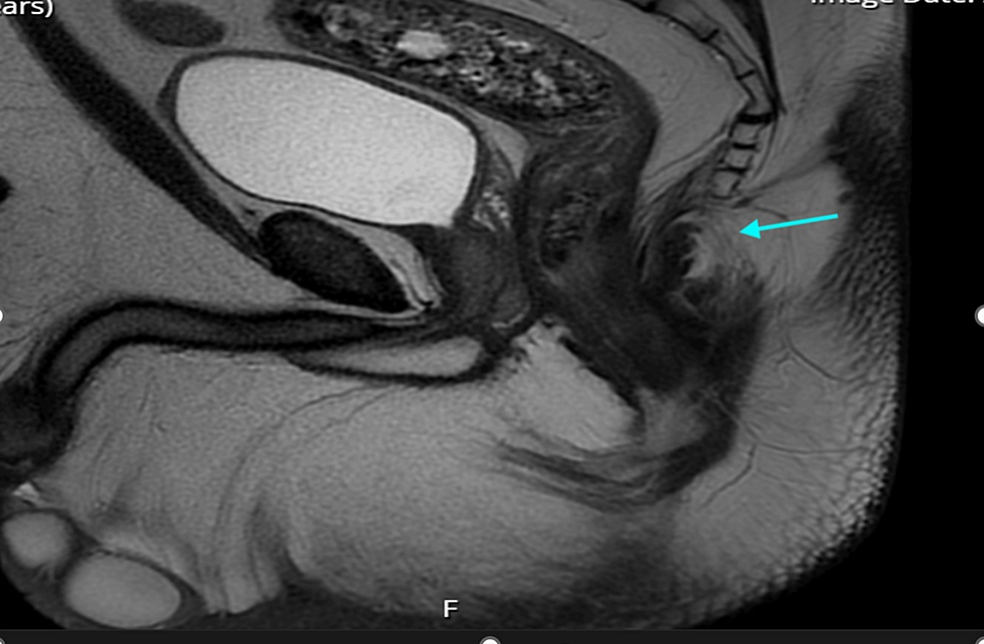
Postoperative (six weeks) MRI. No evidence of residual abscess or fistulae in the perianal and rectal areas.
